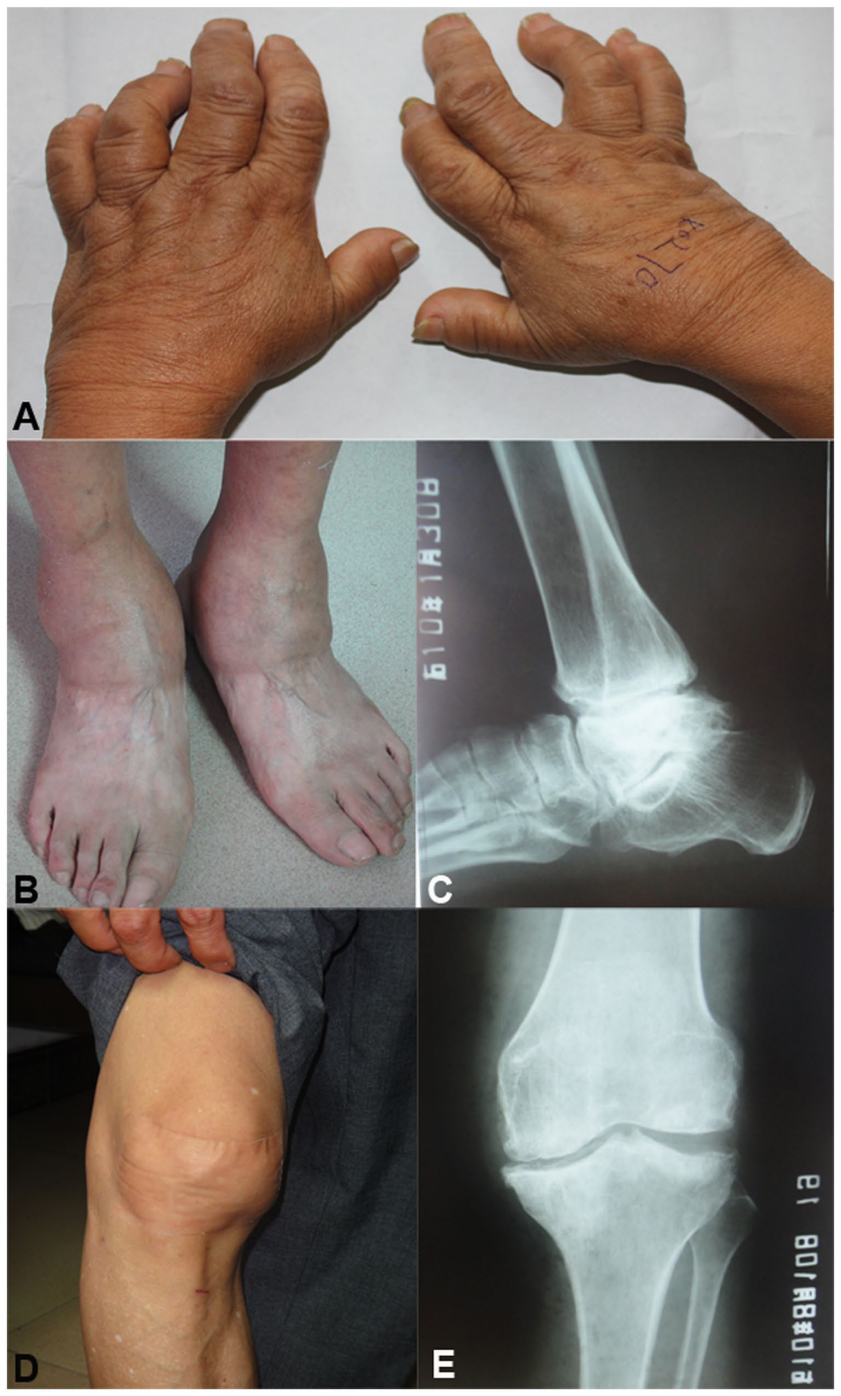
Characteristics of study samples with Kashin-Beck disease. (A) Images of hands; (B,C) image and radiographic image of ankle; (D,E) image and radiographic image of knee.
